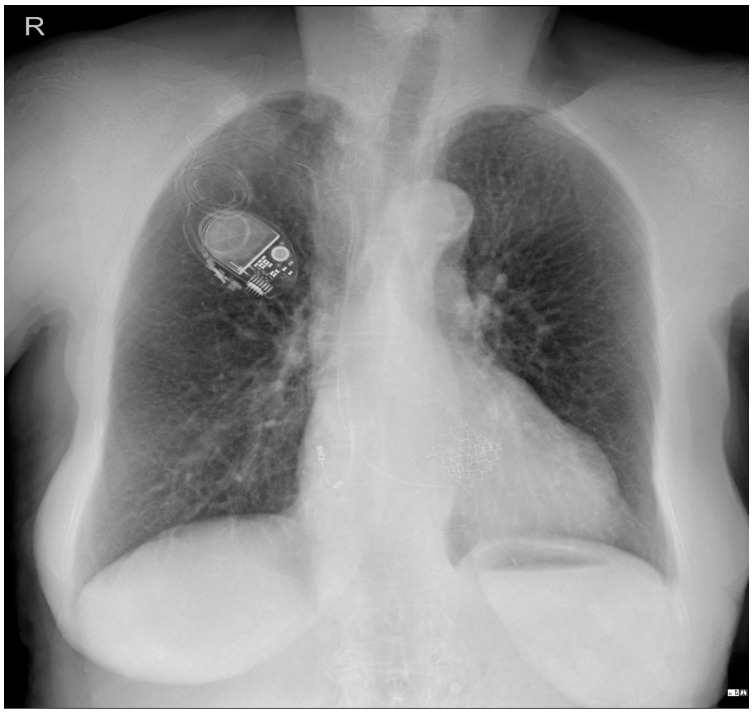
Patient chest X-ray at admission: a cardiac pacemaker is present in the right pectoral region with a ventricular stimulation lead placed on the free wall of the right ventricle and an abandoned atrial lead. There is no apparent lead displacement or fracture. The inferior segment of the left border of the heart is elongated and bulging. An aortic prosthetic valve is present. There are no pleural or pulmonary lesions.
